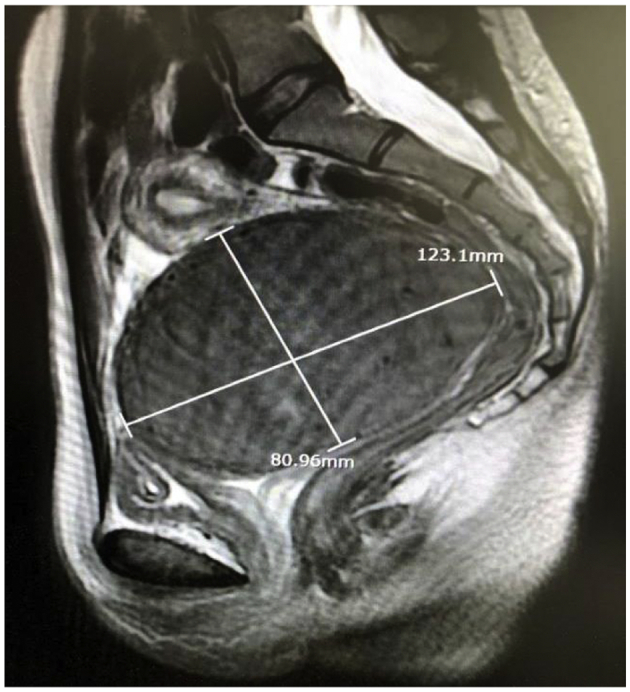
Pelvic magnetic resonance imaging, in sagittal T2-weighted sequence. A large 12 cm leiomyoma of left lateral cervical sidewall, FIGO 8, filling the pelvis.
